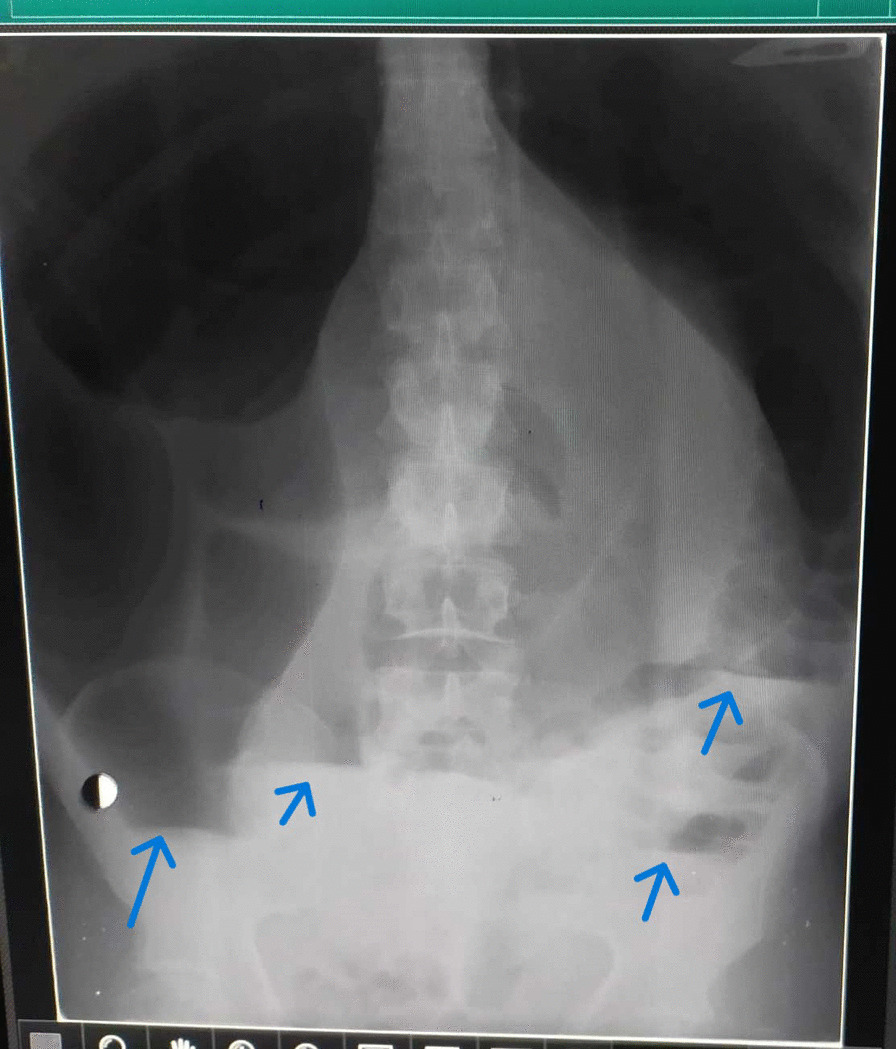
Abdominal X-ray showing extremely dilated colon and air–fluid levels (blue arrows)
